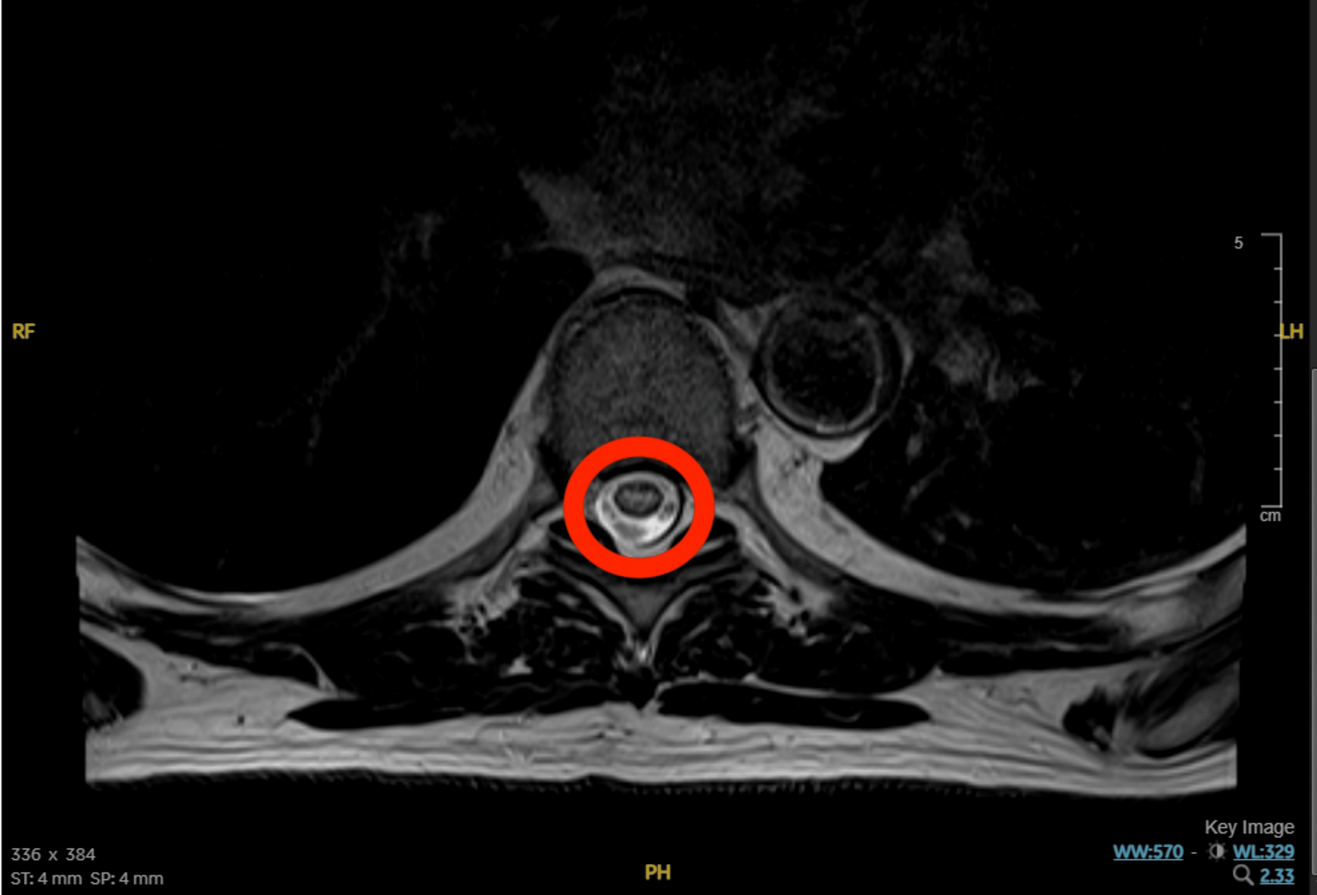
MRI image showing "owl's eye" sign (circled in red).
